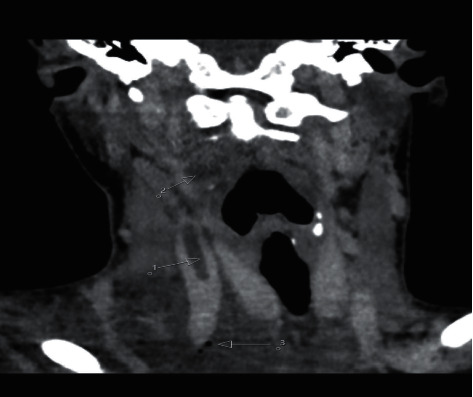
Neck computed tomography scan, coronal reconstruction, shows a thrombus in the right internal jugular vein (1), hypodense fluid collection in the ipsilateral parapharyngeal space (2), and gas inclusions in the supraclavicular region (3).
